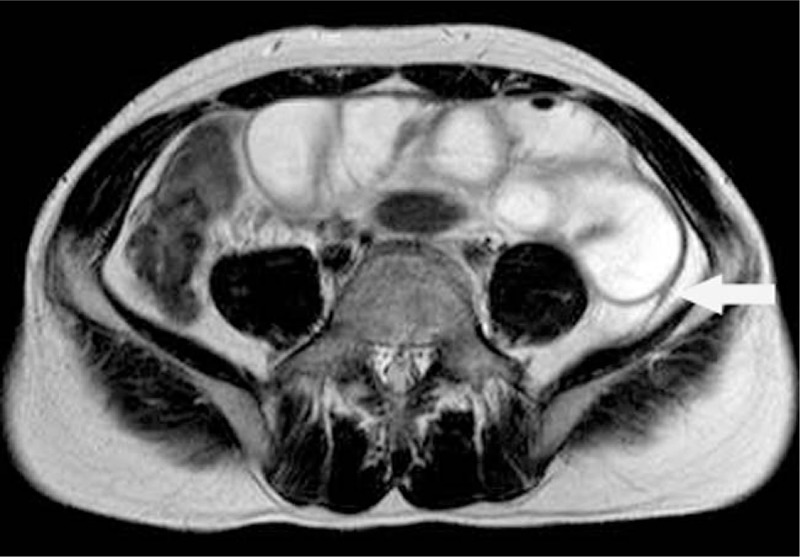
Abdominal axial T2WI MR images show dilated and tortuous intestine, with linear low signal encapsulated (arrow). MR = magnetic resonance.
